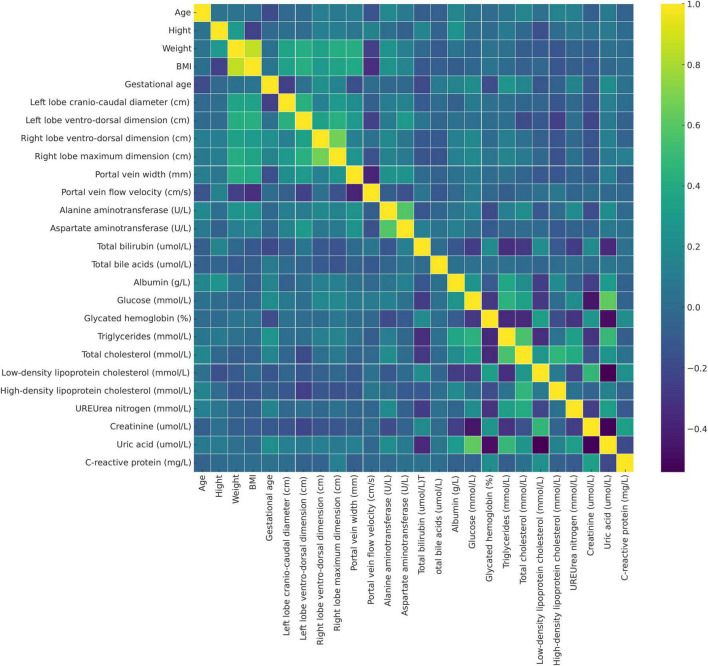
Heatmap displaying the correlation matrix of various clinical and Doppler ultrasonography parameters in the study population, including pregnant women with acute fatty liver of pregnancy (AFLP) and healthy controls. Each cell represents the correlation coefficient between two variables (purple, indicating negative correlation and yellow, indicating strong positive correlation). This heatmap helps identify potential interdependencies among variables and the relationship of these markers with AFLP pathology.
